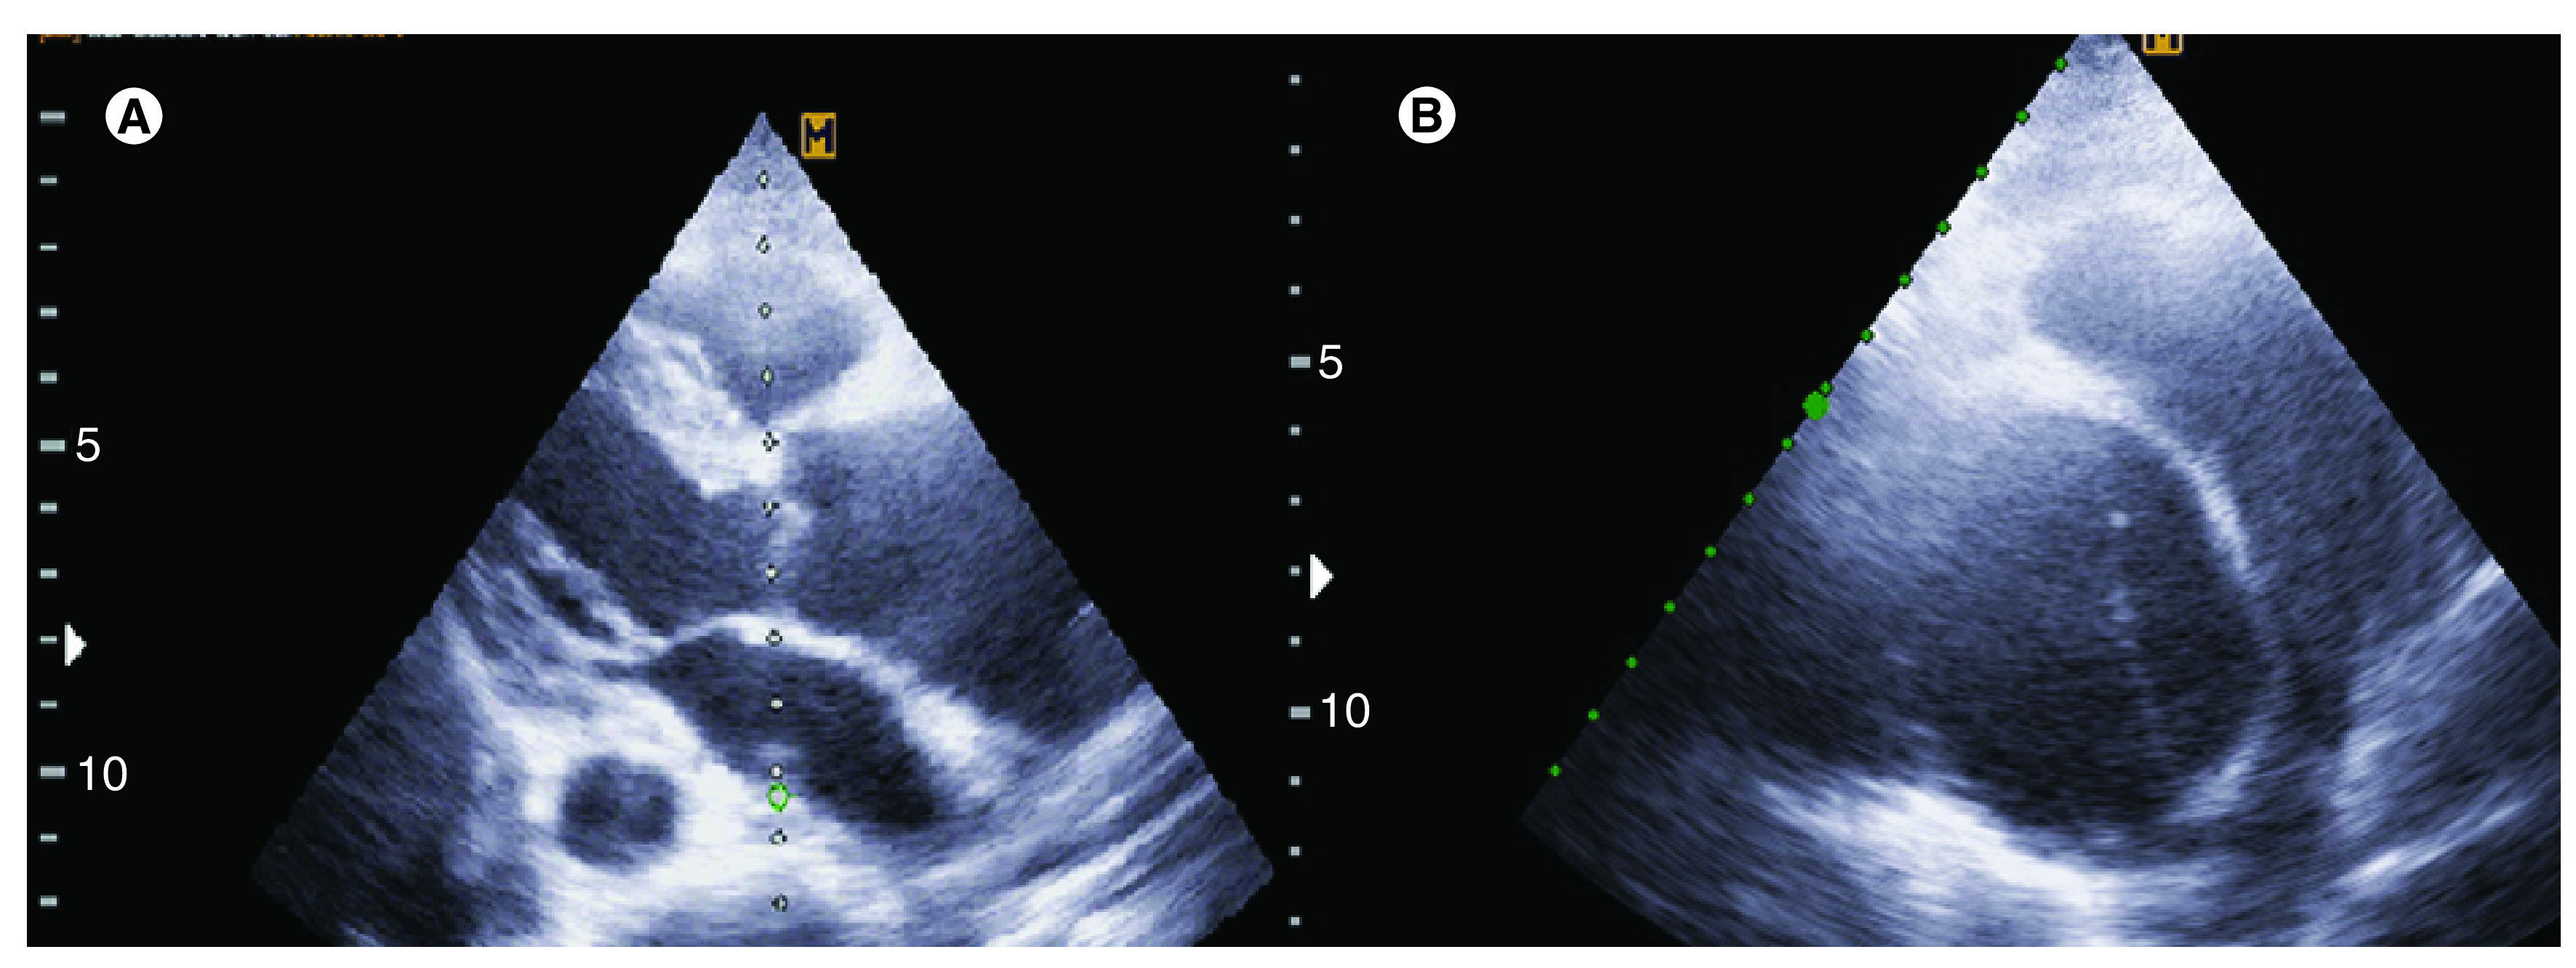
Transthoracic echocardiography. (A) Dilated ascending aorta in parasternal long-axis view and (B) intimal flap is seen in ascending aorta in short-axis view.
